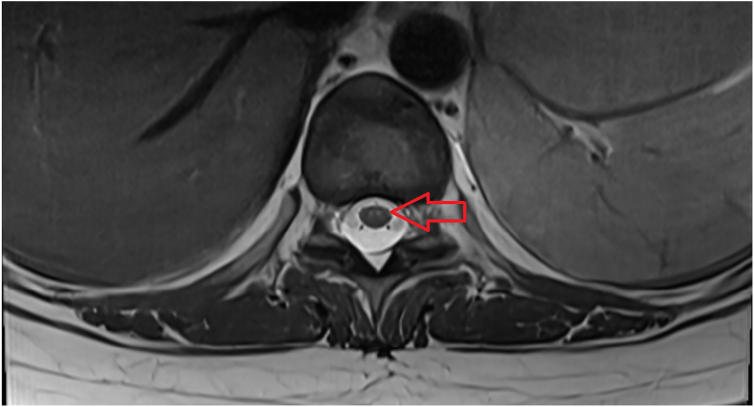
Axial T2-weighted magnetic resonance imaging of the T9–T11 intervertebral disc showing two areas of infarction.
